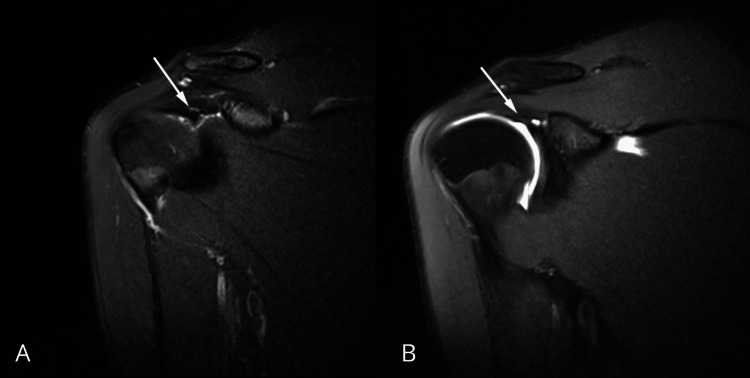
Coronal MRI images of the right shoulder A: proton density fat-suppressed sequence showing preserved integrity of the coracohumeral ligament and normal rotator interval anatomy. B: T1-weighted fat-suppressed MR arthrography image with intra-articular contrast confirming intact coracohumeral ligament, without evidence of rotator interval or bicipital pulley system injury
